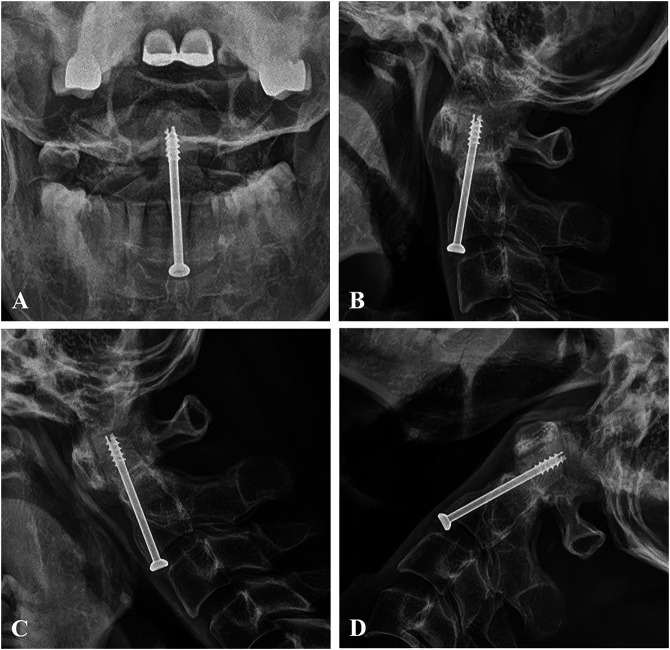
At 2 years after surgery, follow-up lateral radiograph (A) and open mouth view (B) showing fusion of the type 3 dens fracture with correction of angulation. Flexion and extension lateral radiographs (C and D) showing no instability of the C1-2 joint.
